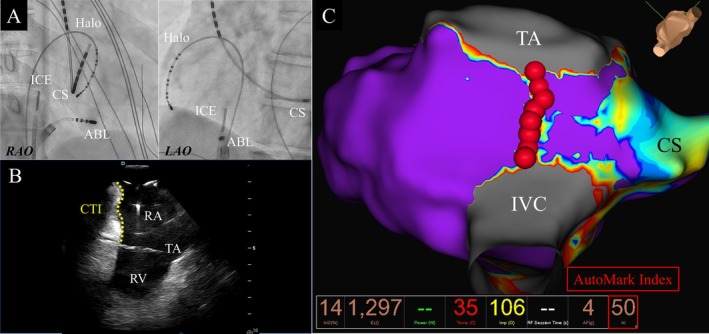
Multimodality for cavotricuspid isthmus ablation. (A) Fluoroscopic images in right and left anterior oblique. (B) Intracardiac echocardiography of cavotricuspid isthmus anatomy. (C) Voltage mapping and geometry from the tricuspid annulus to the inferior vena cava in an inferior view. The target of the AutoMark index was set at 50 (red tags) for the whole segment. AI, Automark index; ABL, ablation catheter; CS, coronary sinus; CTI, cavotricuspid isthmus; TA, tricuspid anulus; ICE, intracardiac echocardiography; IVC, inferior vena cava; RA, right atrium; RV, right ventricular; SVC, superior vena cava.
